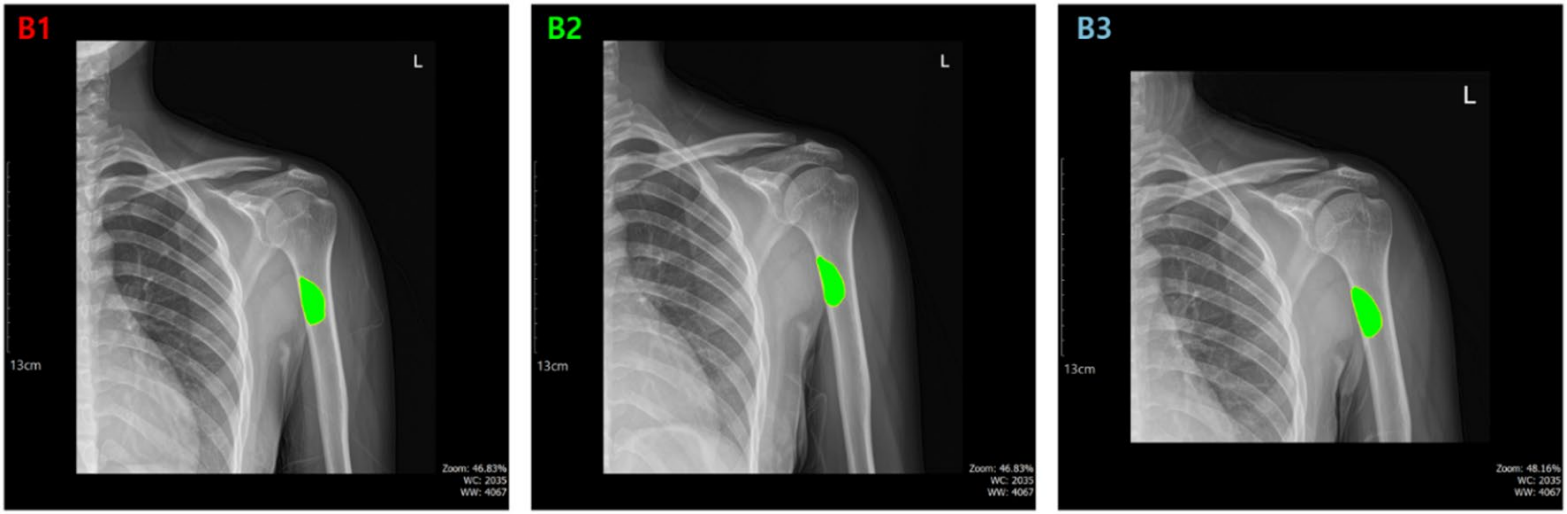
Sequential X-ray images of the humerus from a single patient, taken over a one-year period, with segmented regions corresponding to BBT areas.
